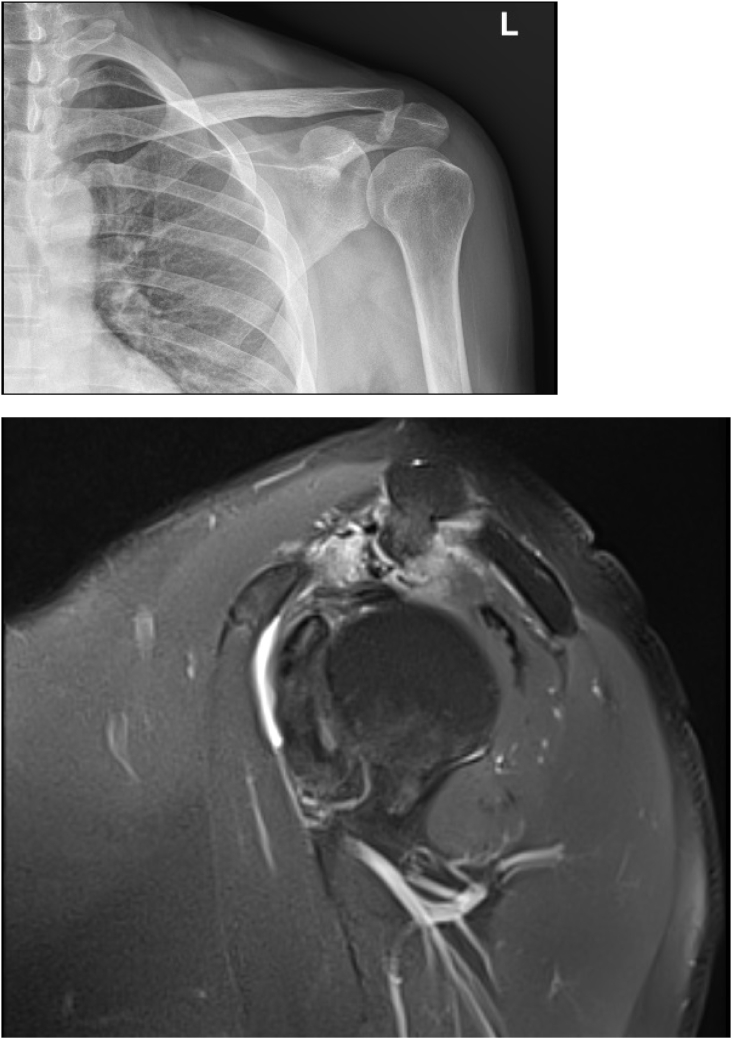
X-Ray Pre-operative showes distal clavicle exostotic bone and MRI revealed distal clavicle exostotic bone causing pressure effect on rotator cuff.
